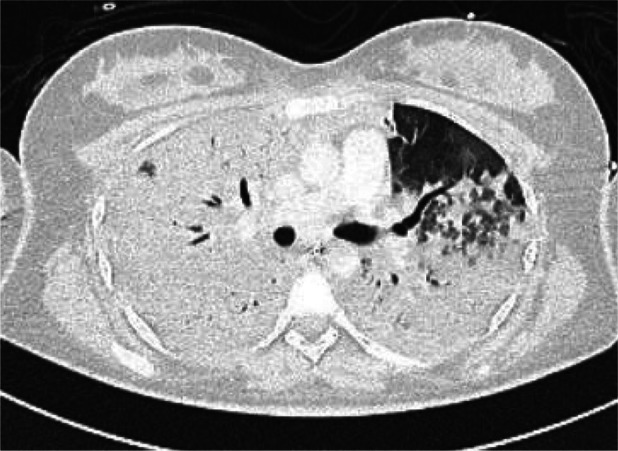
Axial Chest CT scan with IV contrast (lung window). There is diffuse, near‐complete parenchymal opacification of both lungs, with air bronchograms noted.
